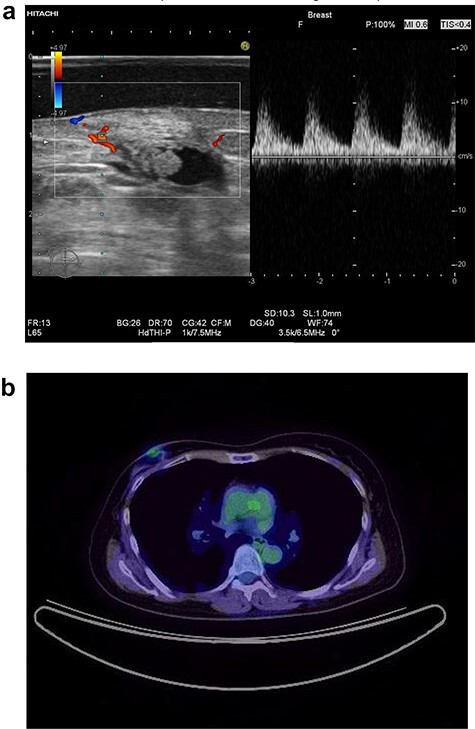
Ultrasound of the right breast in case 1 revealed a mass-like lesion 16.6 × 10.0 × 4.9 mm (a), and PET-CT showed that the right breast mass had an SUV max of 2.7 (b).
